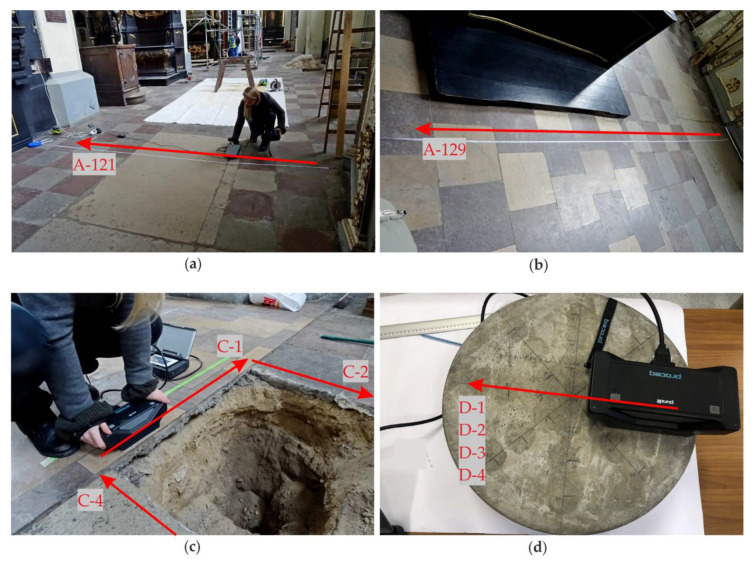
Ultrasonic measurements: (a,b) along traces A-121 and A-129 in the south aisle; (c) along edges of the trial pit; (d) along circular slabs.
